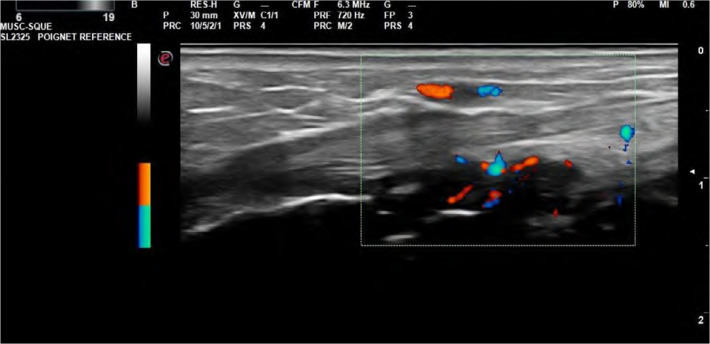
Ultrasonography of the hands showing positive Doppler synovitis (grade 3) of the right wrist.
